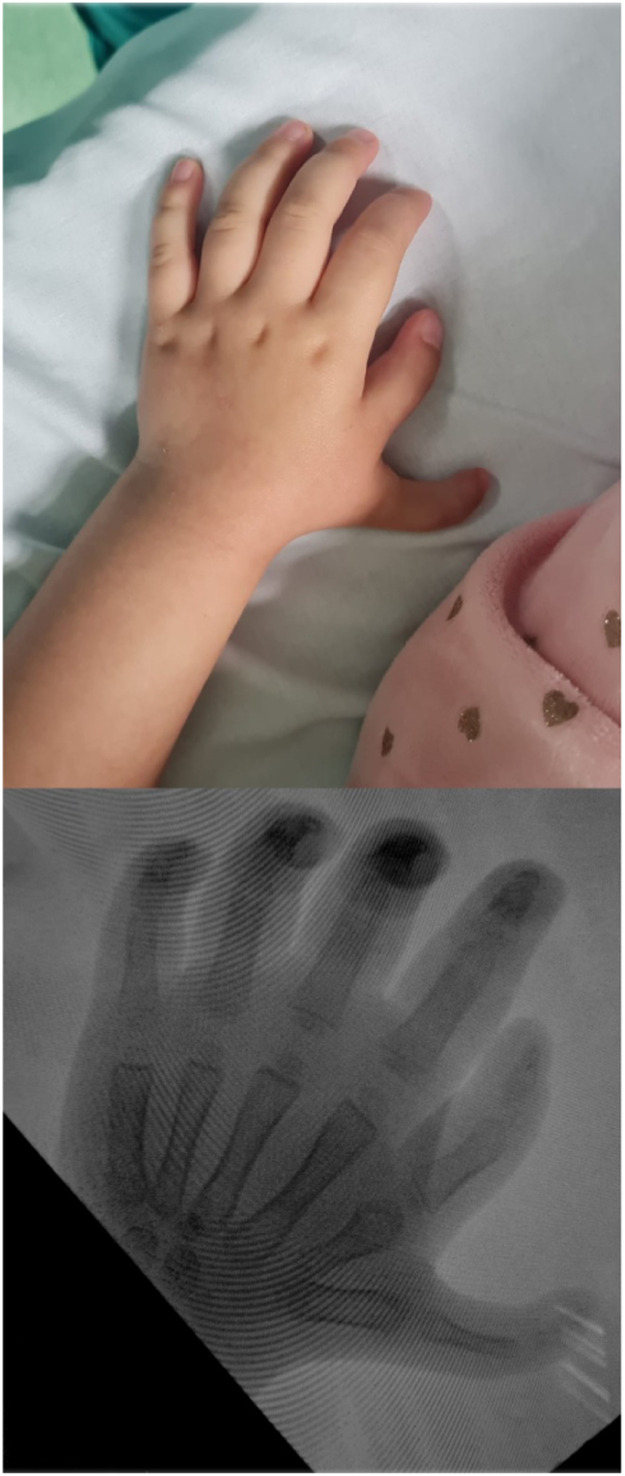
Wassel VI of the thumb is evident on a preoperative plain radiograph.
